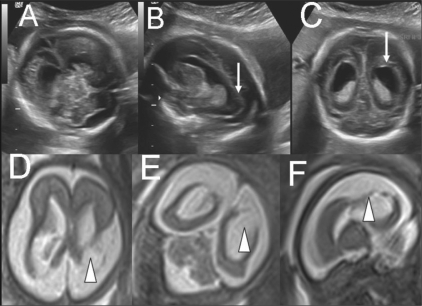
Single live gestation at 24 weeks. USG images (A-C) in different planes show ventriculomegaly with prominent occipital horns (arrows) and widened subarachnoid spaces. The possibilities of neuronal migrational and an atrophic disorder were considered. T2W axial (D), coronal (E), and sagittal (F) MRI images show bilateral symmetric total neuroparenchymal loss in the parieto-occipital watershed regions (arrowheads) but no clefting; this is suggestive of an ischemic etiopathogenesis rather than a neuronal migrational disorder. The couple opted for termination of pregnancy. They declined an autopsy
